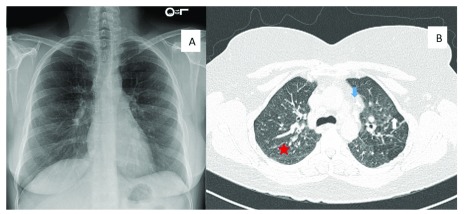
A chest radiograph and high-resolution computed tomography from the same patient are depicted. (
